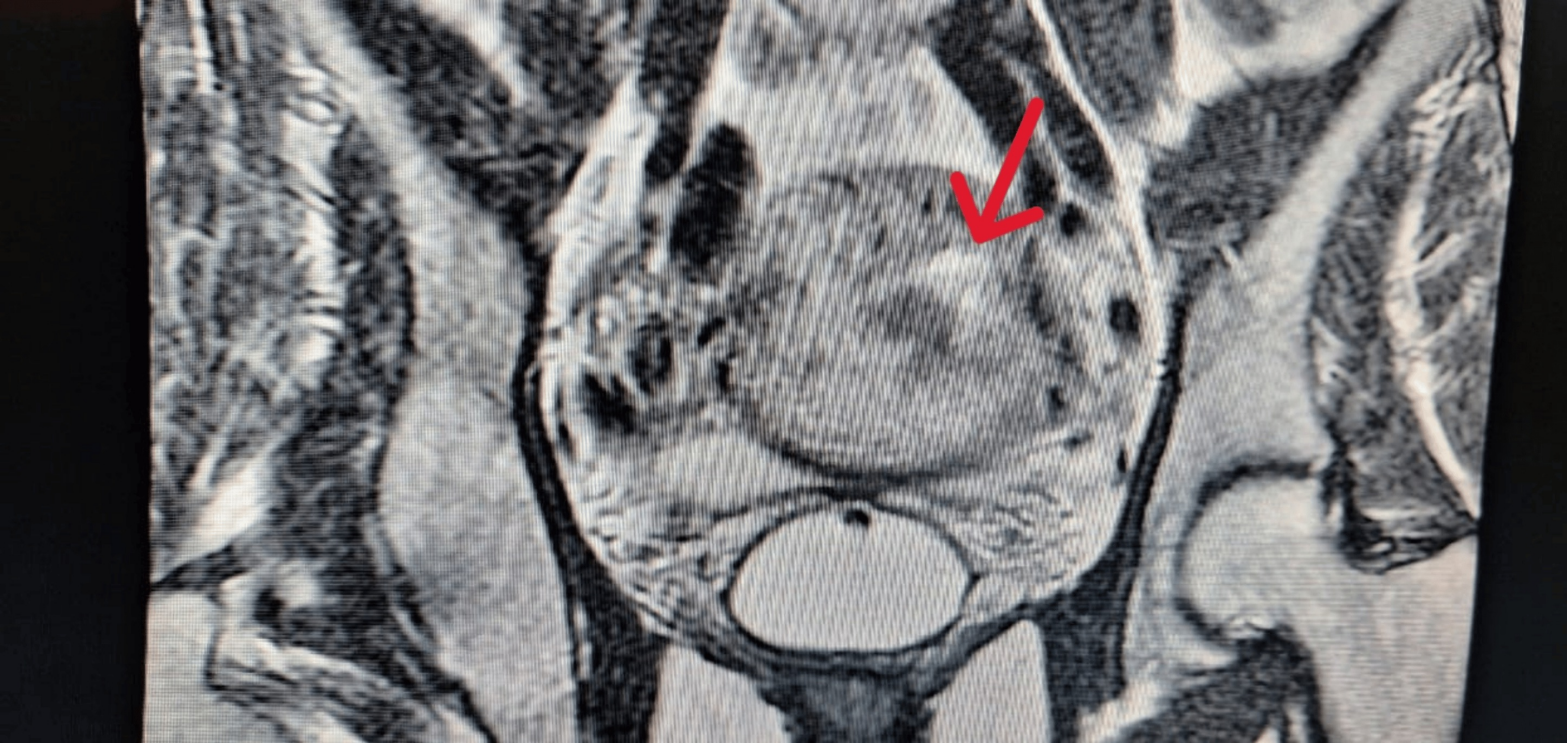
MRI coronal T2-weighted image showing heterogeneous hyperintensity of the mass (red arrow).
